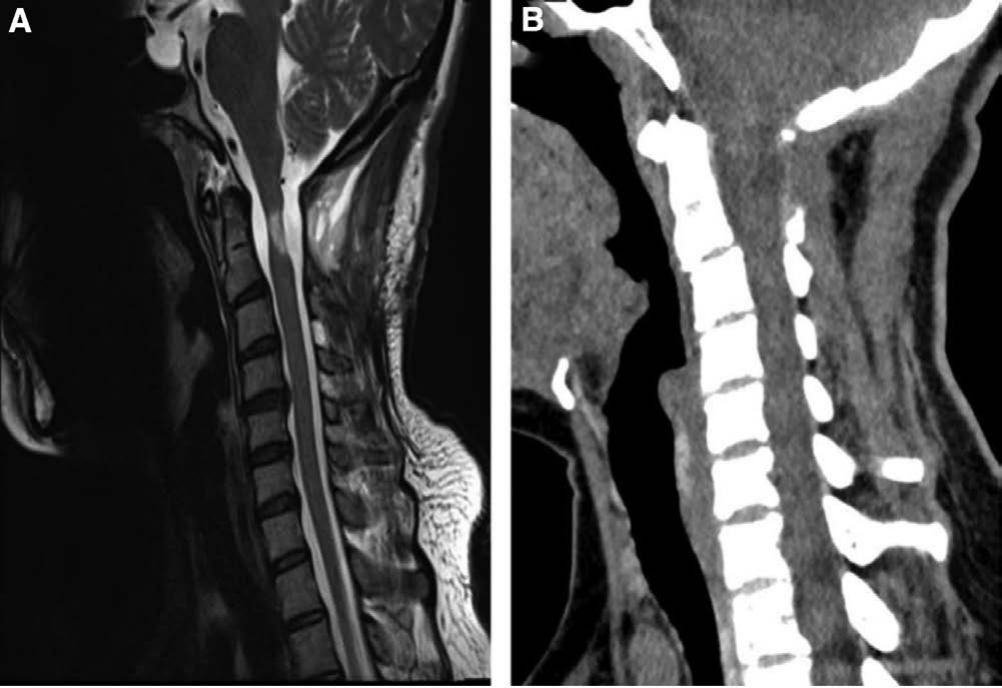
(A) MRI on the fifth day after surgery. (B) CT after 3 months of follow-up.
